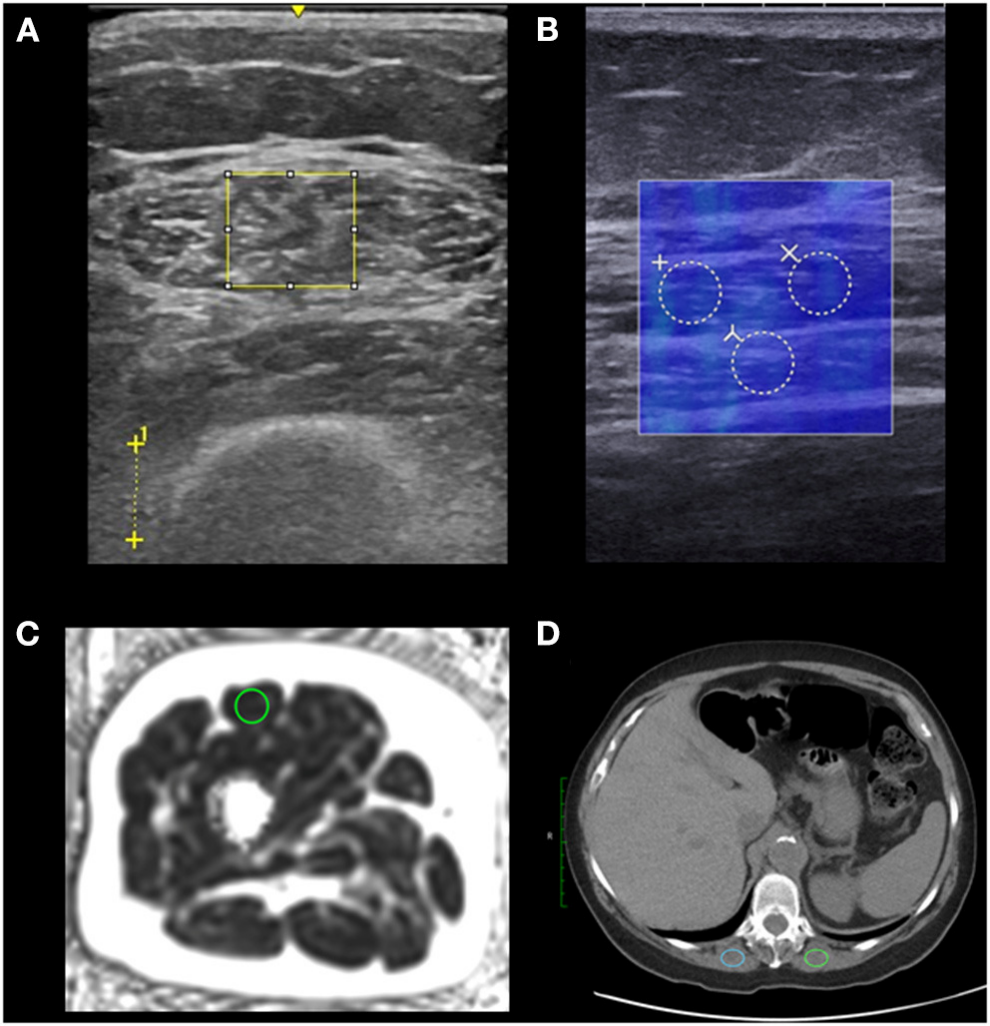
Regions of interest (ROIs) used to analyze myosteatosis. We placed ROIs in the rectus femoris of the right leg using ultrasound and MRI (*n* = 30), and ROIs in the erector spinae muscles using CT for older adults undergoing treatment (*n* = 10). We placed the largest possible rectangular ROIs to assess echo intensity on brightness-mode ultrasound and used the distance between crosses to indicate 1 cm to set image scale for measuring tissue thicknesses in post processing (A). We placed three 3 mm diameter circular ROIs which were averaged to assess shear wave speed measured by shear wave elastography. The shear wave speed measurements (in blue) are coregistered with a brightness-mode image to identify tissue locations (B). We placed a 1 cm^2^ circular ROI to assess proton density fat fraction using MRI (C). We placed 2 ovular 2 cm^2^ ROIs which were averaged to assess the muscle density using CT of older adults undergoing treatment (D).
